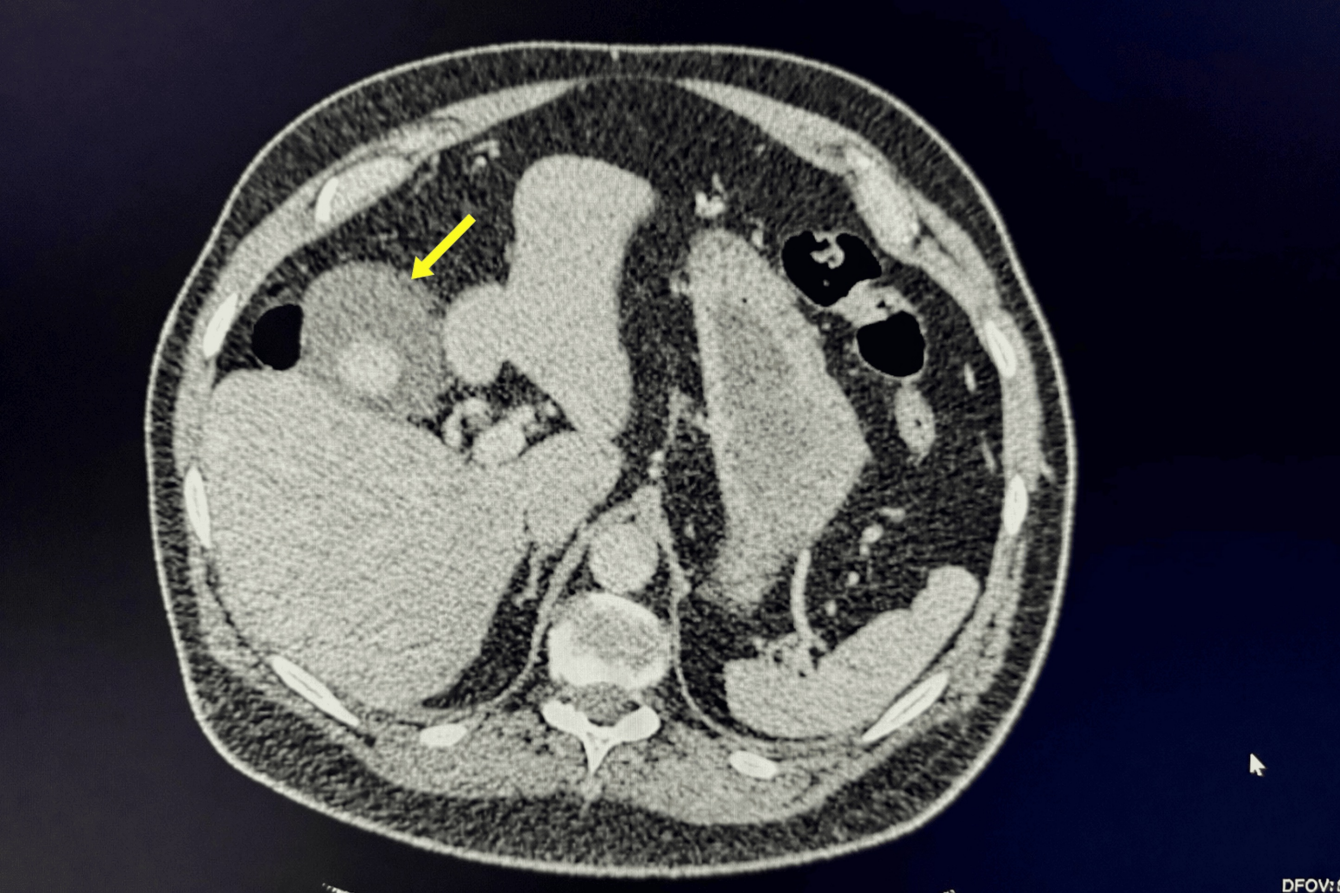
CT scan of the abdomen and pelvis showing inflamed gallbladder with gallstone
